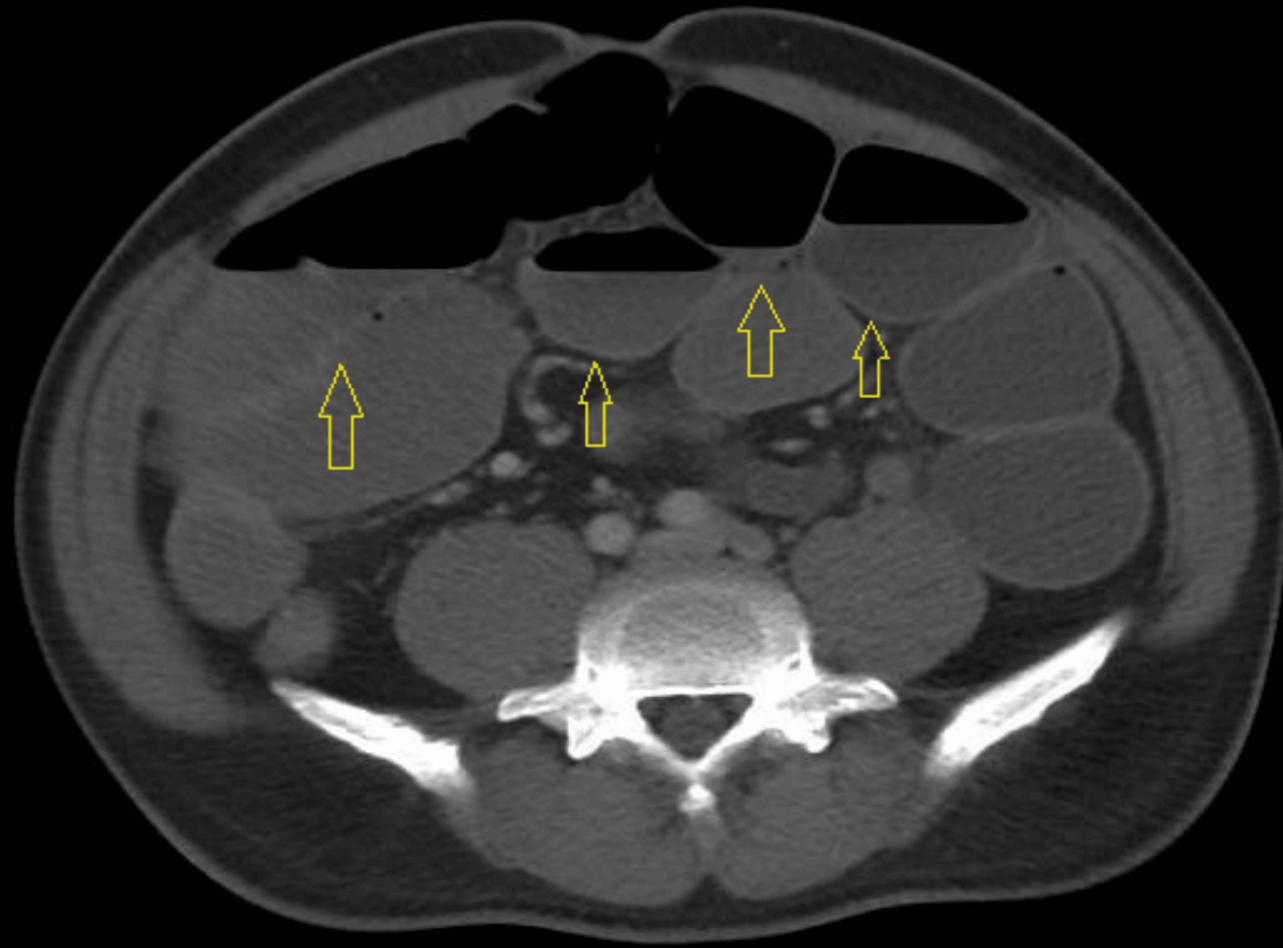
Axial contrast-enhanced CT scan of the abdomen demonstrating diffusely dilated, fluid-filled small-bowel loops with mild caliber change in the distal ileum (arrows), without a clear transition point. The presence of distal colonic gas suggests a partial distal small bowel obstruction versus ileus. No pneumatosis, portal venous gas, or free intraperitoneal air is identified.
